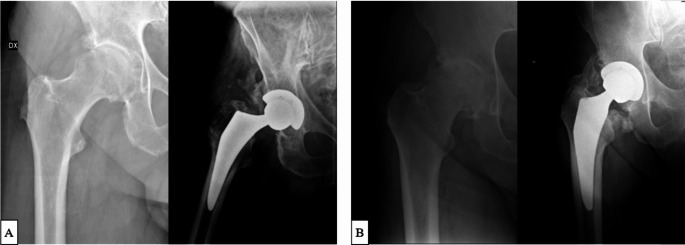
Pre-operative and 6 months post-operative X-rays. (A) Brooker 3 HO after DAA approach. (B) Brooker 4 HO after DLA approach
